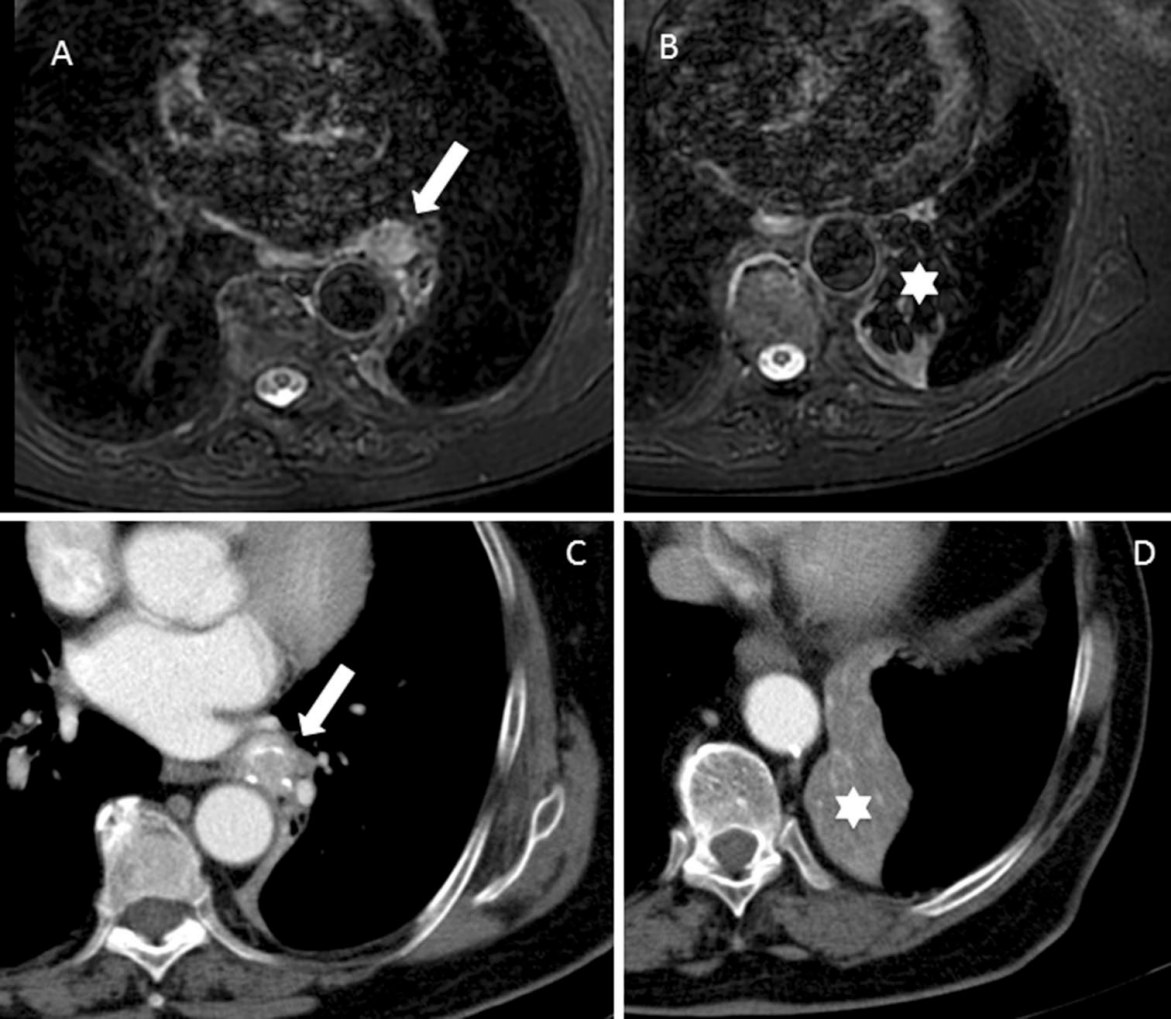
Comparison between STIR sequence and CT for differentiation between atelectasis and NSCLC. a, b STIR sequences where the tumor (arrow) and the associated atelectasis (asterisk) can be clearly identified and differentiated. However, in c, d CT images, the distinction between atelectasis and tumor is less evident
